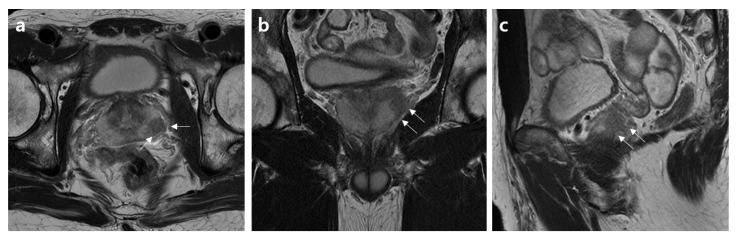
MR images of a 75-year-old patient with an extensive extraprostatic extension (EPE). (a) Axial, (b) coronal, and (c) sagittal T2-weighted MR images show capsular bulging or irregularity associated with a left peripheral zone mid-posterior lesion (arrows). The basic clinical findings included a serum PSA level of 15.3 ng/mL, a prostate volume of 37 mL, a positive biopsy core of 40%, clinical stage of T2b, biopsy grade 3, and intermediate D’Amico risk. PI-RADS v2.1 scores and Mehralivand EPE grades on multiparametric MRI were 5 and 2, respectively, according to both radiologists.
